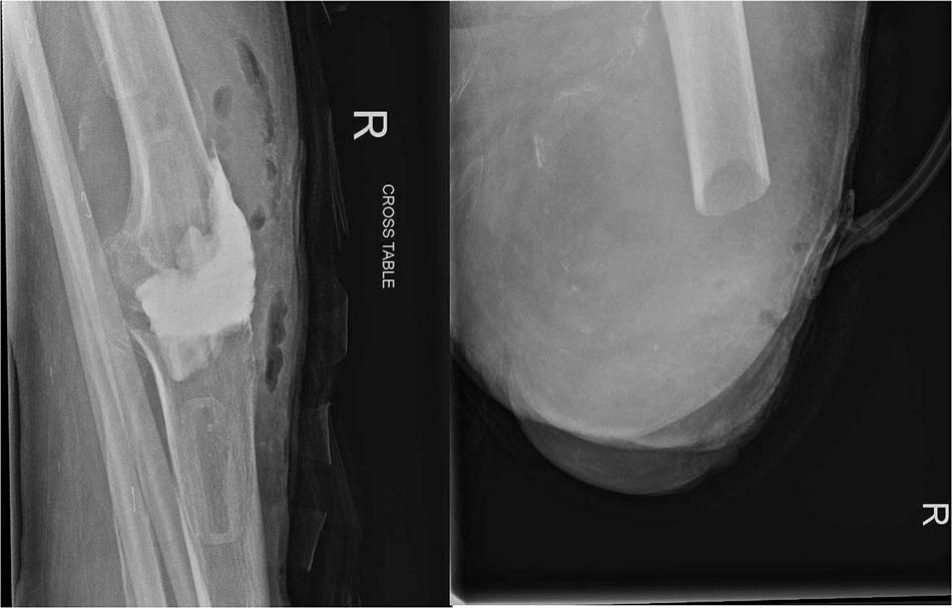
On the left X-ray of the knee after removal of prosthesis and insertion of antibiotic-impregnated cement spacer and on the right X-ray of the stump following amputation.
